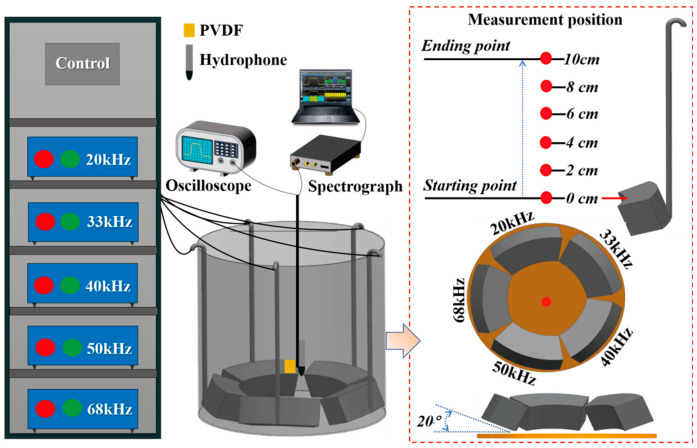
Multi-frequency arc-shaped flat-plate ultrasonic device with time–frequency domain monitoring system.
